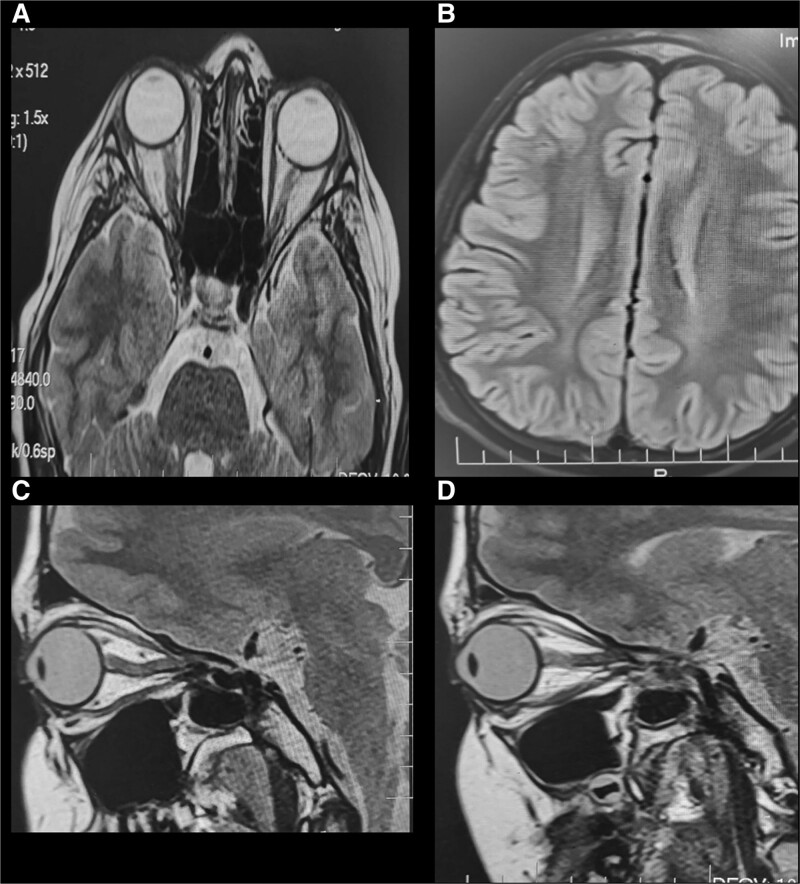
(A) MRI/T2 FLAIR showed evidence of abnormal areas of peripheral enhancement, and increased optic nerve tortuosity, along the course of right optic nerve. (B) Normal brain parenchymal. No lesions (C and D) with some asymmetric right more than left (B). MRI = magnetic resonance imaging. FLAIR = fluid-attenuated inversion recovery.
